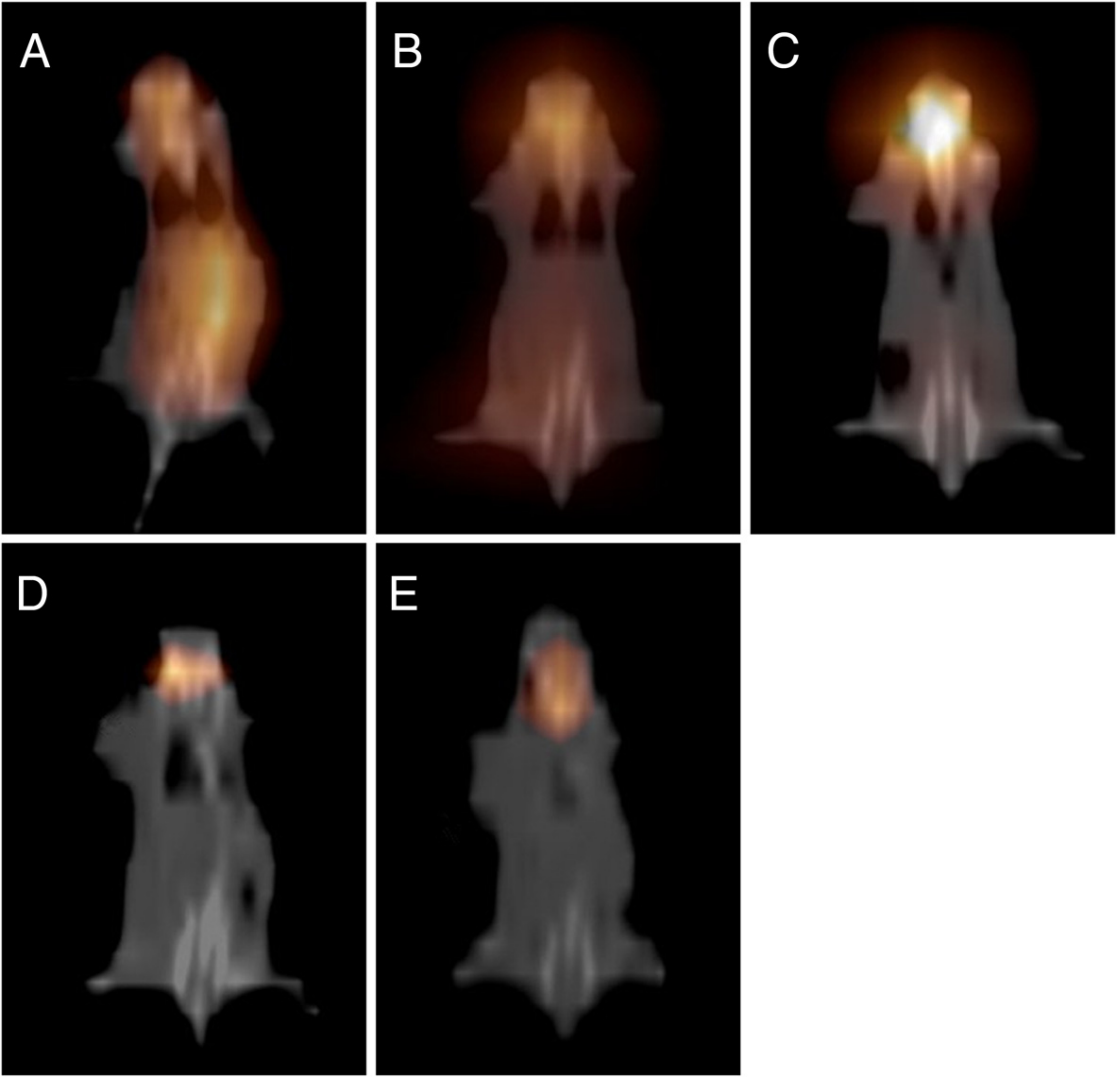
The results of SPECT imaging at 1 (A), 6 (B), 24 (C), 48 (D) and 72 h (E) after the administration of [^
